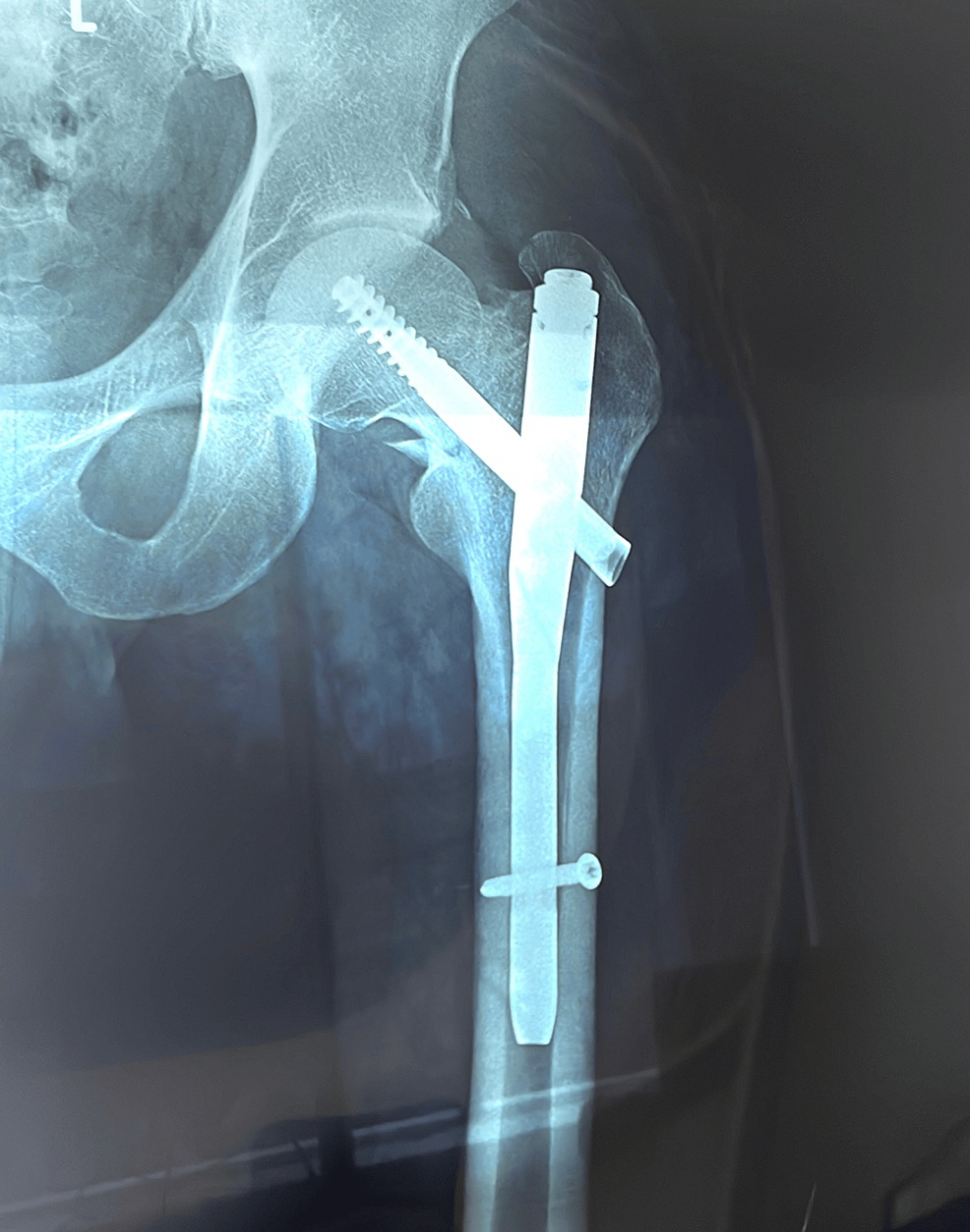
Anteroposterior Radiograph of the Left Femur Post-Internal Fixation with Osteosynthesis
